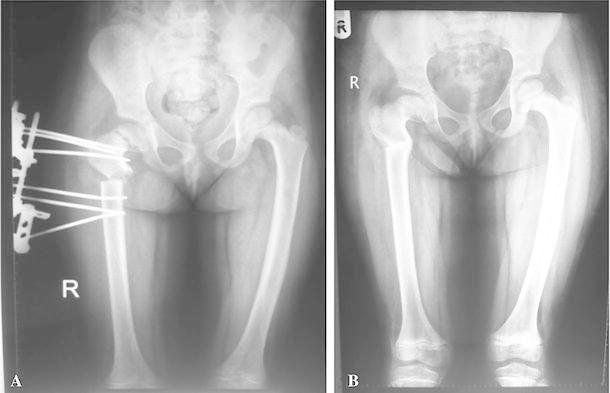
a X-ray for postoperative follow-up of osteotomy after acute correction, b healed osteotomy with correction of neck-shaft angle
